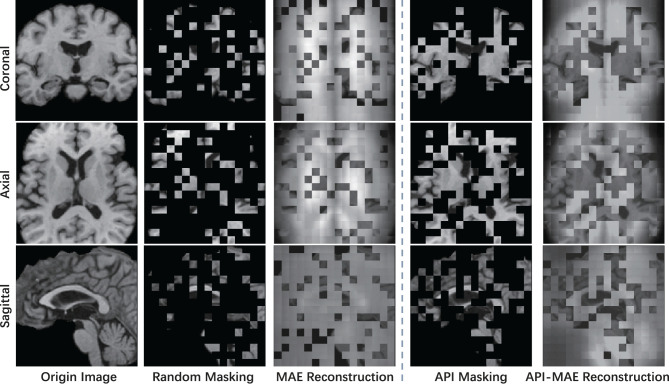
The visible example of masking images and the reconstruction results of MAE and API-MAE. The five columns represent the origin brain T1 MR image, the random masking strategy used in MAE, the masking image generated from API token sampling, and the reconstruction results of API-MAE, respectively.
